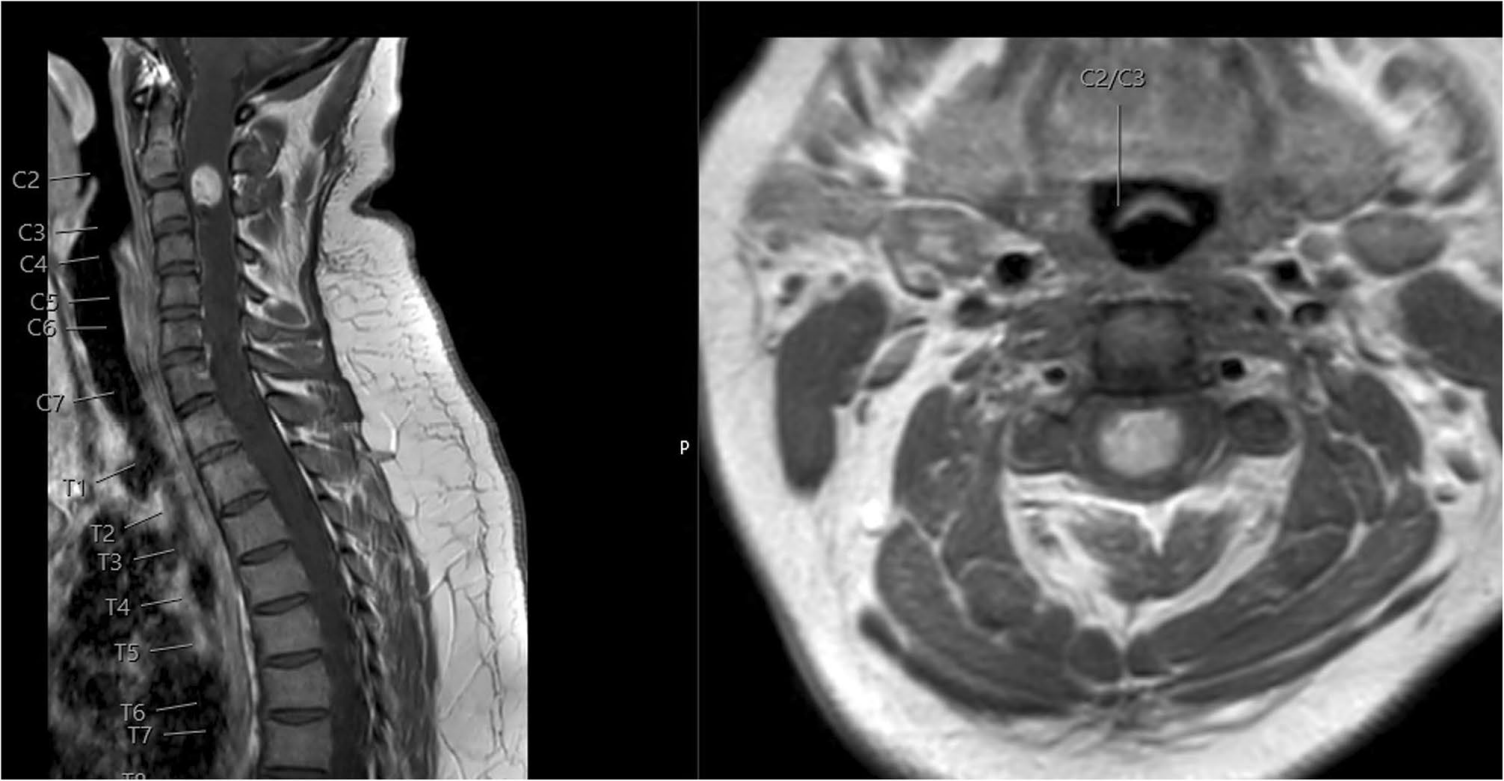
T1-MRI scan with contrast enhancement showing a contrast-enhancing lesion reaching from C2 to C3 with extensive vascularization
